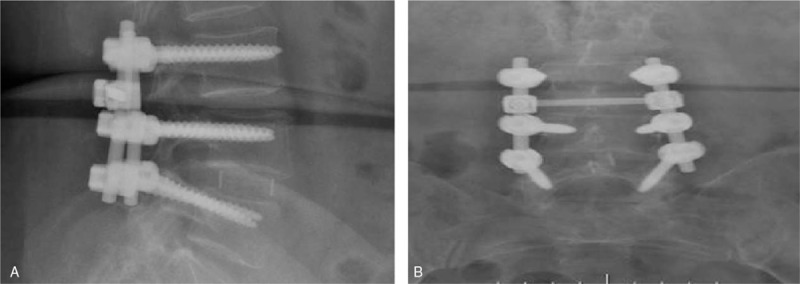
Postoperative X-ray lateral and AP view of open lumbar discectomy. (A) Postoperative X-ray lateral view of open lumbar discectomy with pedicle screw fixation of the lumbar spine at the level of L3-L5. (B) Postoperative X-ray AP view of an open lumbar discectomy with pedicle screw fixation of the lumbar spine at the level of L3-L5.
